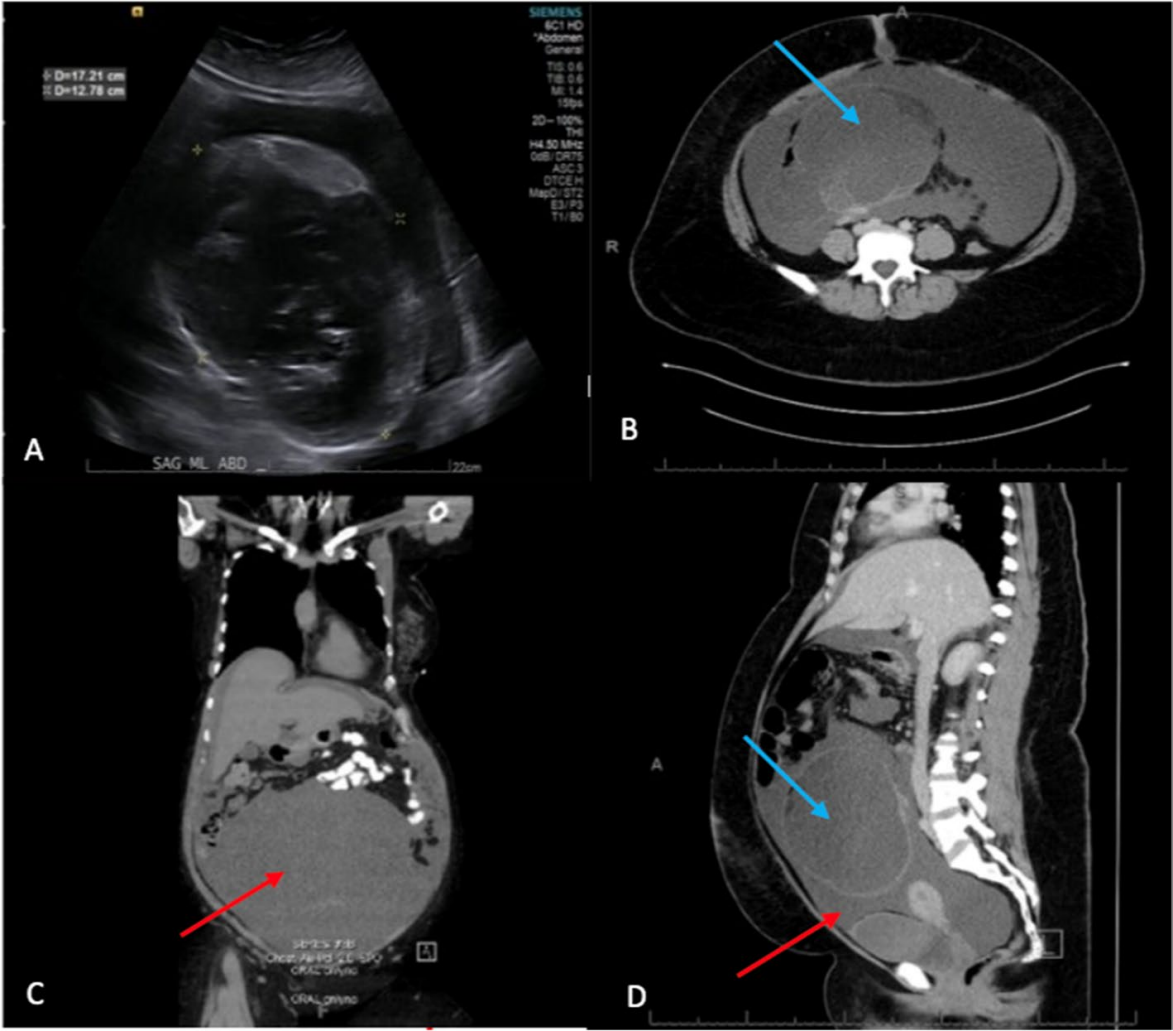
A Ultrasound images of the 18-cm mass. B–D Contrast-enhanced perioperative abdominal CT scan of the 18cm mass in the lower abdominal cavity (B axial, C coronal, D sagittal). The imaging revealed a moderately large volume of mucinous ascites (red arrows) and a large right ovarian mass (blue arrows) concerning for cystic teratoma vs malignancy
